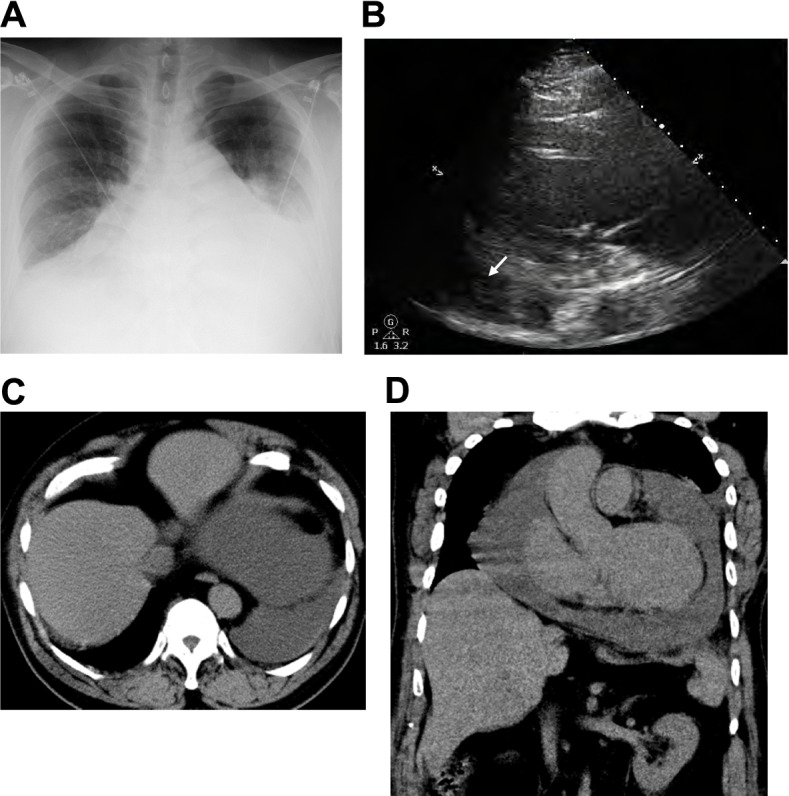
A Pleural effusion was observed in chest X-ray. B A large amount of pericardial fluid was observed in echocardiography (arrow). C, D A large amount of pleural effusion and pericardial fluid were observed in chest and abdominal computer tomography (CT)
